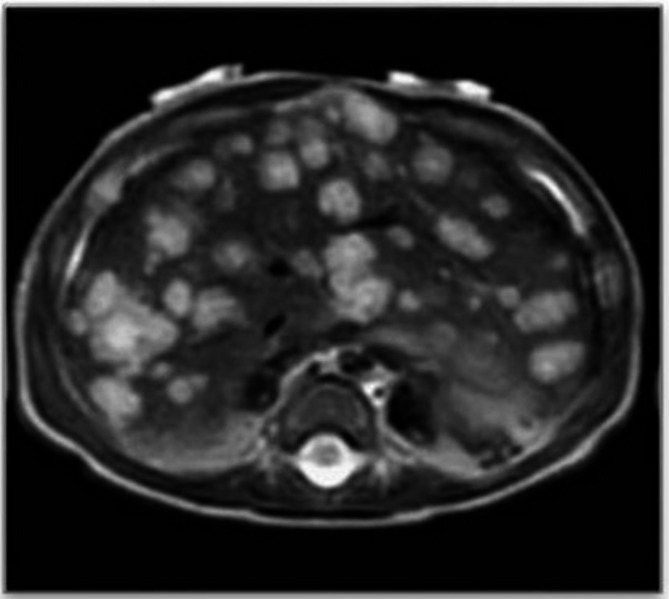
RMI at diagnosis showing hepatomegaly, with diffusely distributed nodules with maximum diameter of 20 mm, hyperintense in T2-weighted images, with nearly total replacement of the hepatic parenchyma, indicative of diffuse IHH
